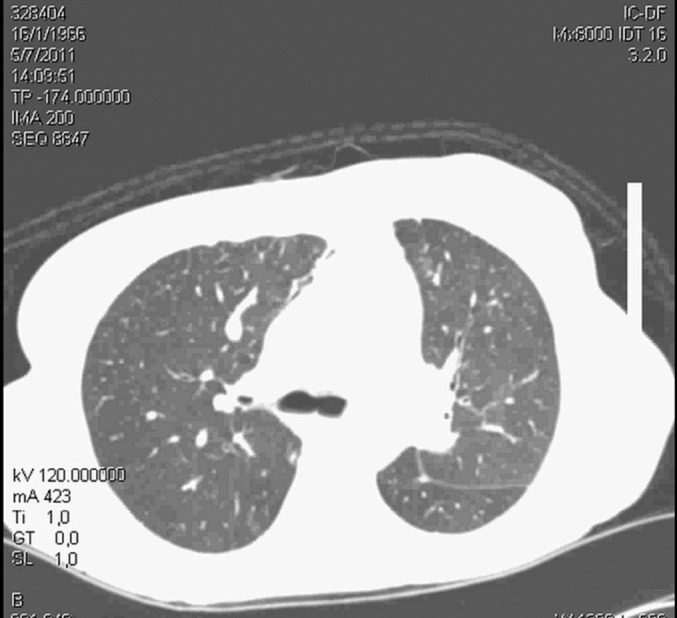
Chest computed tomography performed 25 days after surgery revealing
